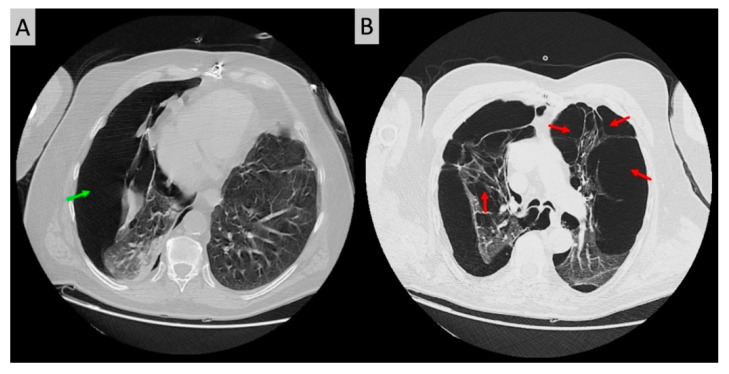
Transverse unenhanced chest CT scan revealing a large right-sided pneumothorax (green arrow) with compressive atelectasis of the right lung and emphysematous changes in both lungs (A); multiple bullae due to the underlying emphysema were noted in the left (red arrows) as well as the right lung in addition to the right-sided pneumothorax (B).
